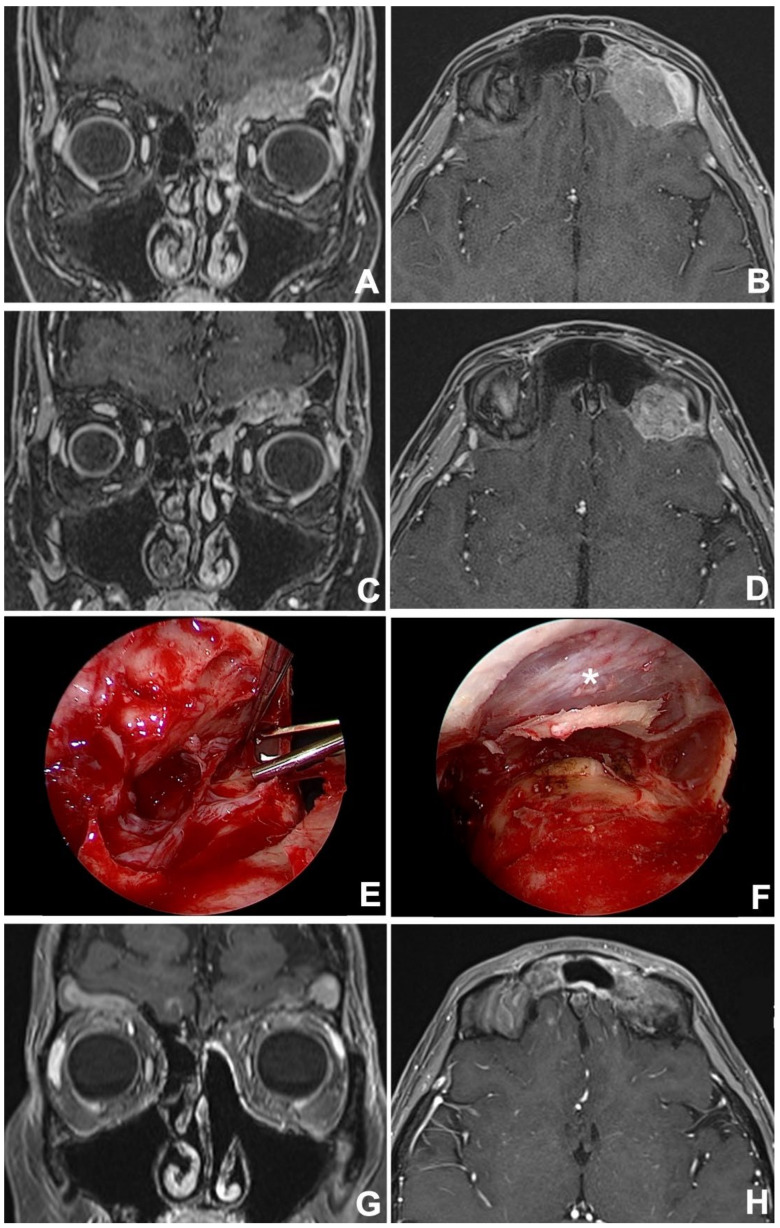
Contrast-enhanced MRI in coronal (A) and sagittal (B) views showing a left frontoethmoidal INI-1 deficient squamous cell carcinoma. After 3 cycles of induction chemotherapy (TPF regimen) an MRI in the coronal (C) and axial (D) view revealed an incomplete response. Tumor persistence was resected via a combined approach: endoscopic transnasal and osteoplastic flap (E), with an exposition of anterior cranial fossa dura (*) which appeared to be free from tumor infiltration (F). At 12 months, postoperative contrast-enhanced MRI in coronal (G) and axial (H) views revealed no local recurrence.
